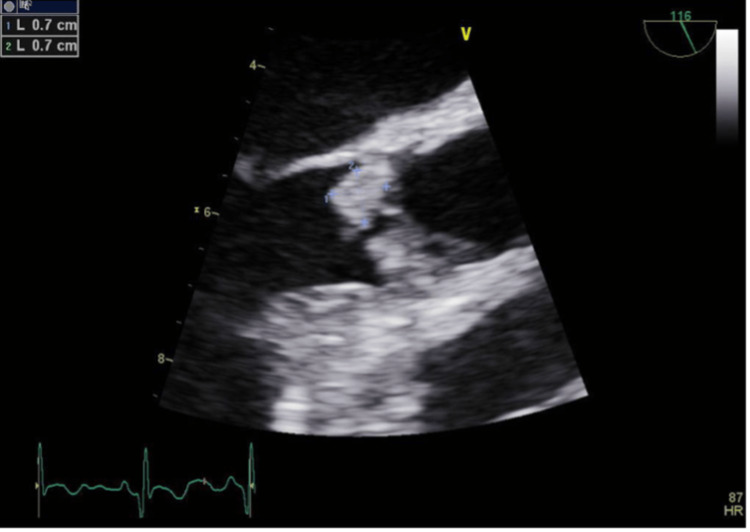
Transesophageal echocardiography (TEE). Transesophageal echocardiography showed large aortic valve vegetation with severe aortic regurgitation.
